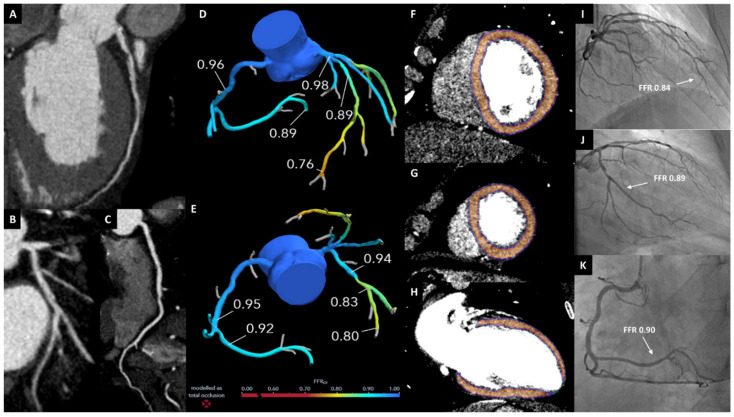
Clinical Case. A 54 y/o male with hypertension, dyslipidaemia and recent onset of atypical chest pain. Panels (A–C): cCTA showing significant stenosis in the proximal LAD (A), moderate stenosis in the proximal LCx (B), and moderate stenosis in the mid RCA (C). Panels (D,E): FFR_CT_ showing grey-zone values in the apical LAD, negative values in the RCA and LCx. Panels (F–H): Static Stress-CTP with short axis views (F,G) and 2-chamber long axis view (H) showing absence of perfusion defects. Panels (I–K): ICA showing moderate stenoses in the proximal LAD (I) and LCx (J), and mild stenosis in the mid RCA (K), all with negative FFR values. cCTA: coronary computed tomography angiography; LAD: left anterior descending; LCx: left circumflex; RCA: right coronary artery; FFR_CT_: computed tomography-derived fractional flow reserve; Stress-CTP: stress computed tomography perfusion; ICA: invasive coronary angiography; FFR: fractional flow reserve.
